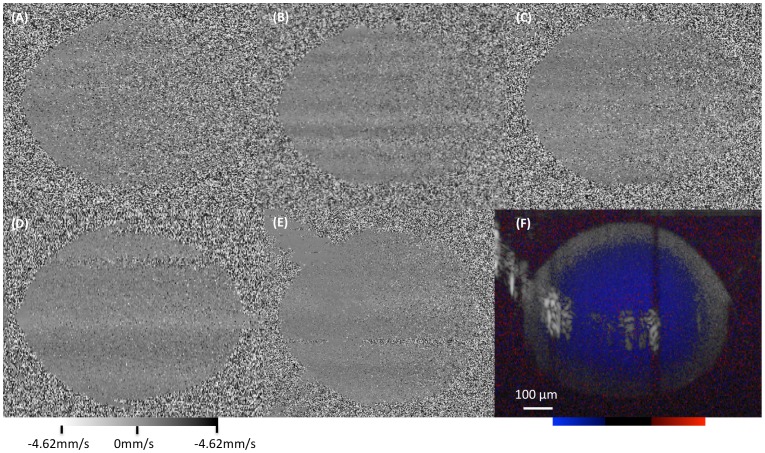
Determining optimal GNR concentration. (A–E) Doppler OCT cross-sectional images of non-flowing gold nanorods (GNR) at (A) 0.3, (B) 0.4, (C) 0.5, (D) 0.7 and (E) 1.0×10^12^ GNRs/mL. The grayscale mapping represents the detected flow towards or away from the OCT scanning beam, centered on 0 mm/s (gray). (F) GNR enhanced saline flowing in a glass tube with a concentration of 1.0×10^12^ GNRs/mL. The color map represents flow towards (red), away (blue) and stationary (black) relative to the OCT scanning beam. (D–F) shows the ability to get Doppler signal with a tube radius of up to 0.32 mm. The red circle corresponds to the outline of the inner surface of the tube. Blue and red corresponded to flow away and towards the probe, respectively. The area of hyper reflective convex arch within F is an imaging artifact.
